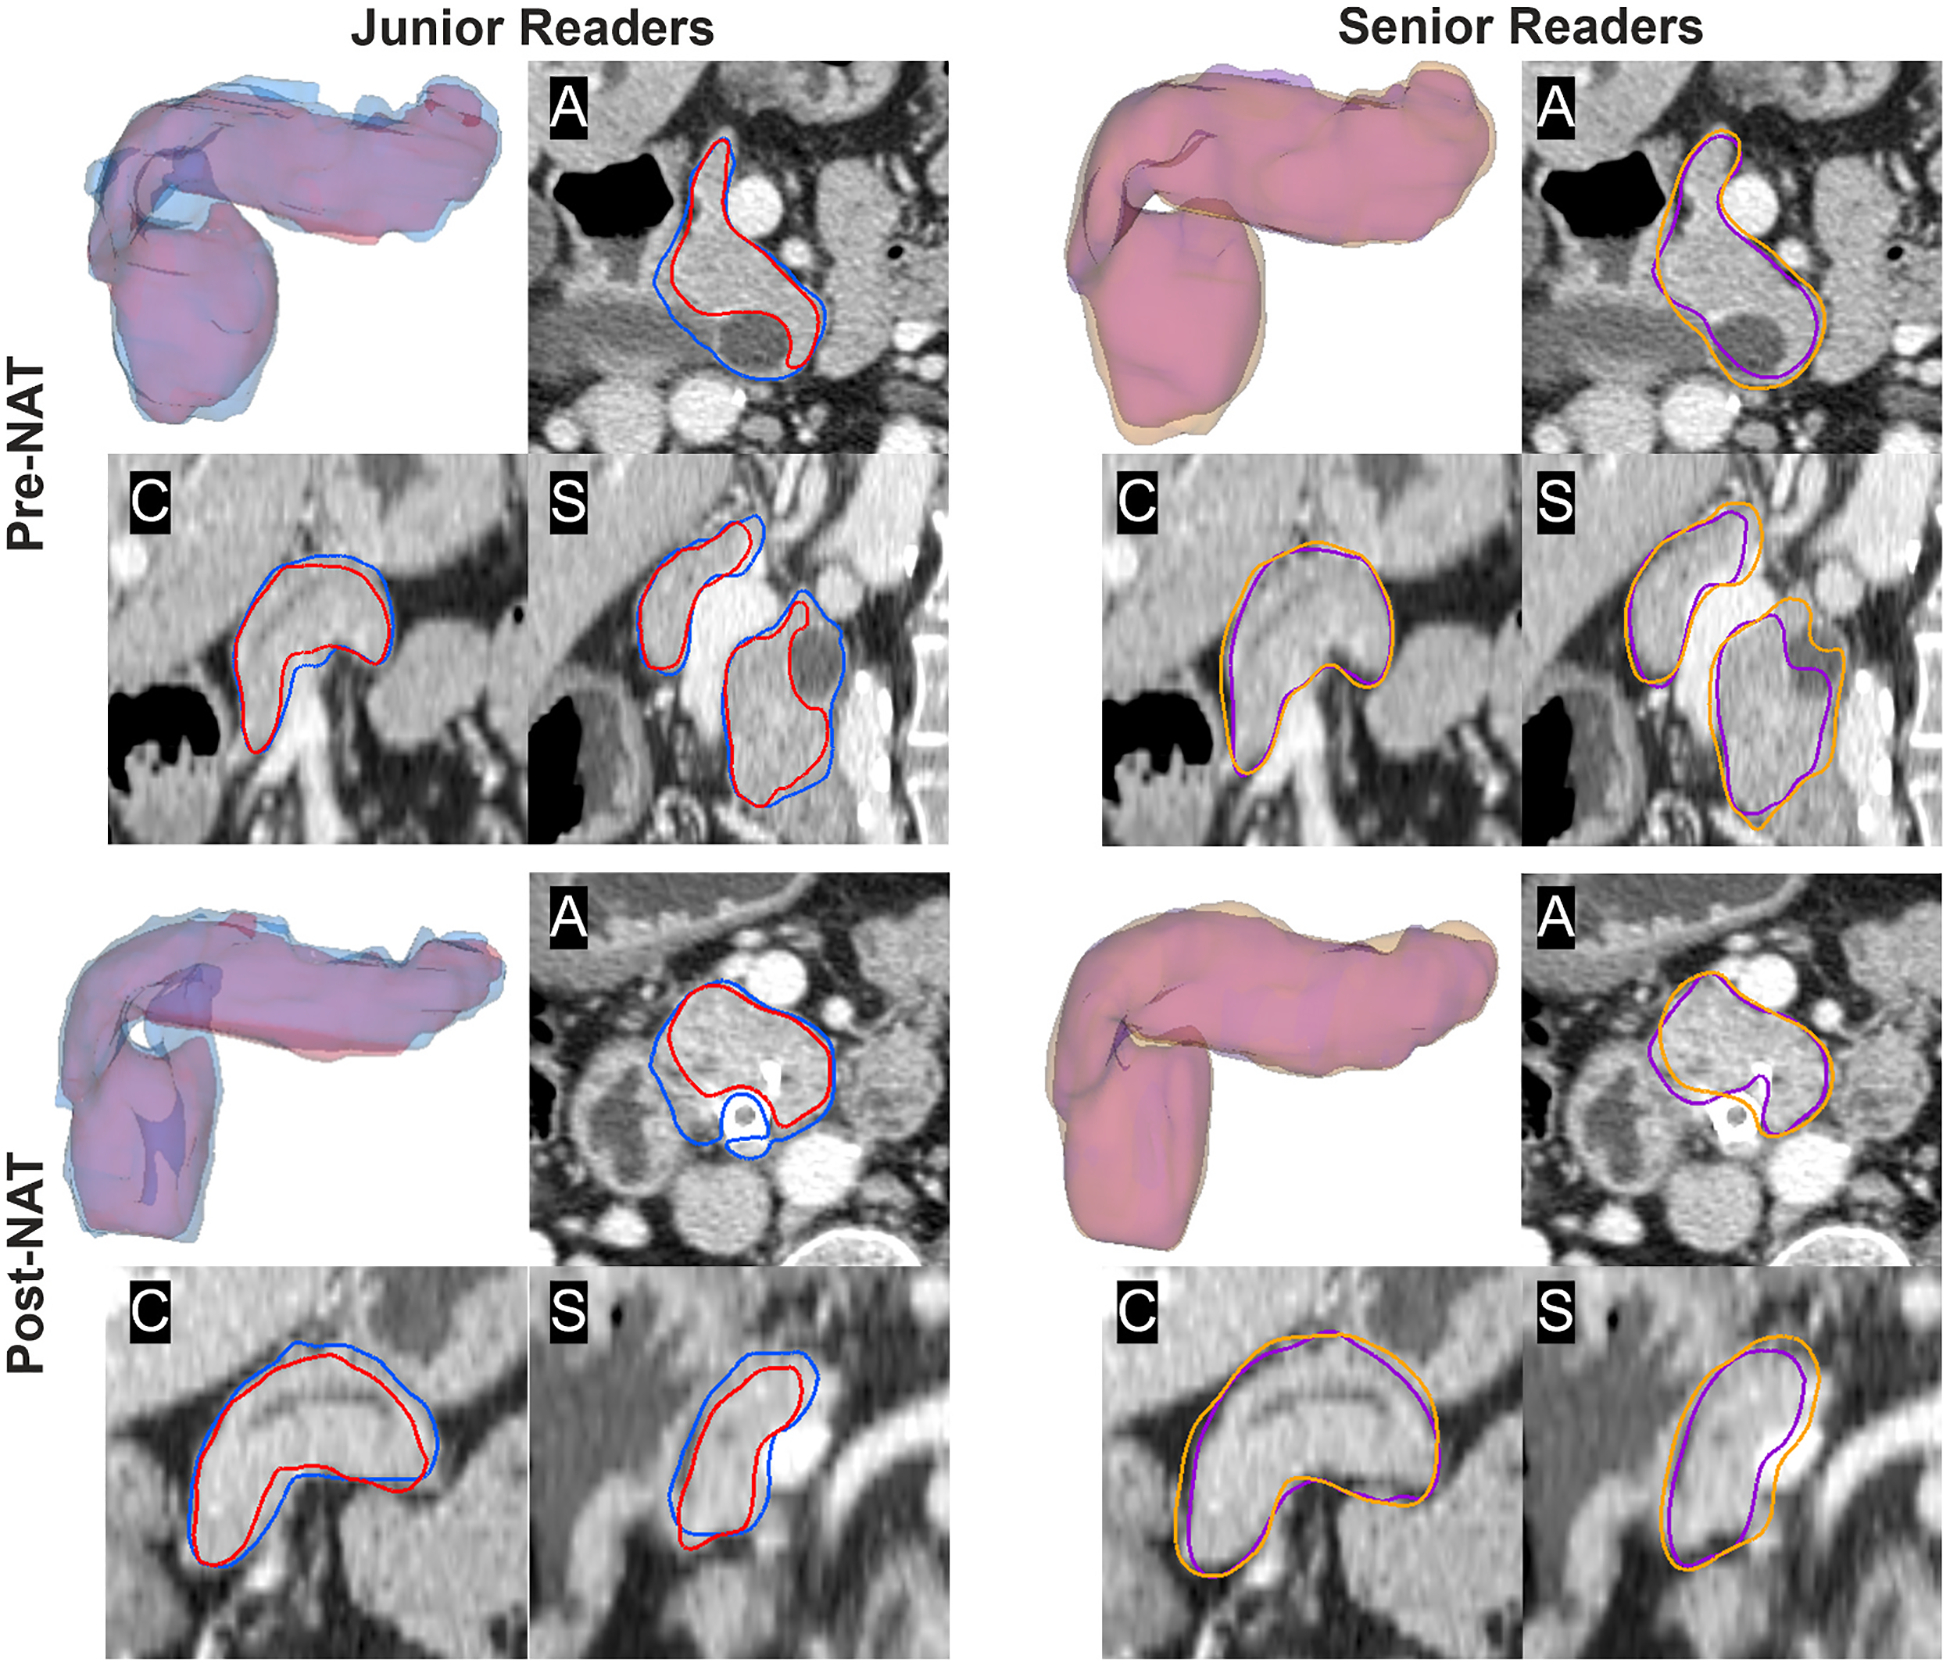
Variability of pancreas segmentations among junior readers (left) and senior readers (right) on pre-NAT and post-NAT CT in one representative patient. (A) axial slice; (C) coronal slice; (S) sagittal slice.
